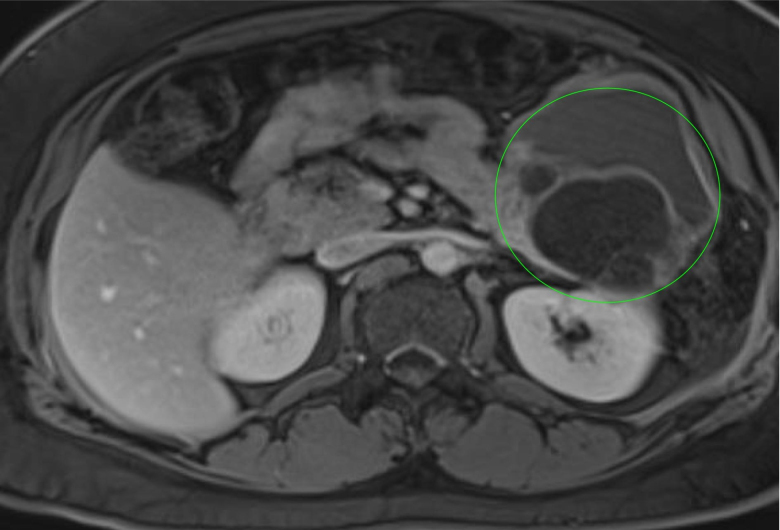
Abdominal magnetic resonance imaging/magnetic resonance cholangiopancreatography: abdominal imaging showing an 8.4 × 12.8 × 16 cm mass abutting the pancreas with moderate volume ascites.
